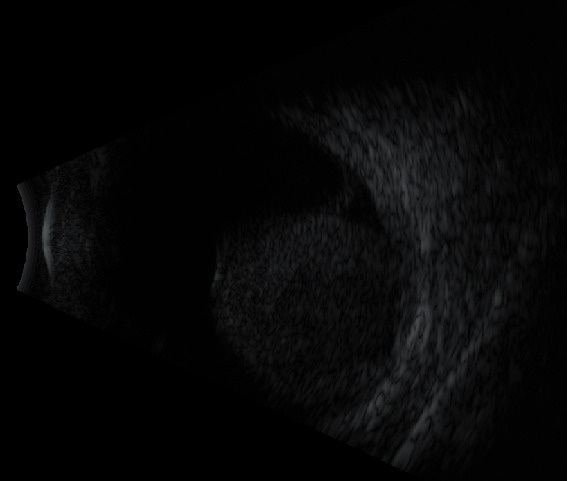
B-scan ultrasonography: large choroidal dome-shaped mass, with neither acoustic hollowing nor calcification, associated with a retinal detachment (subretinal fluid around the mass, without RD extending to the periphery).
